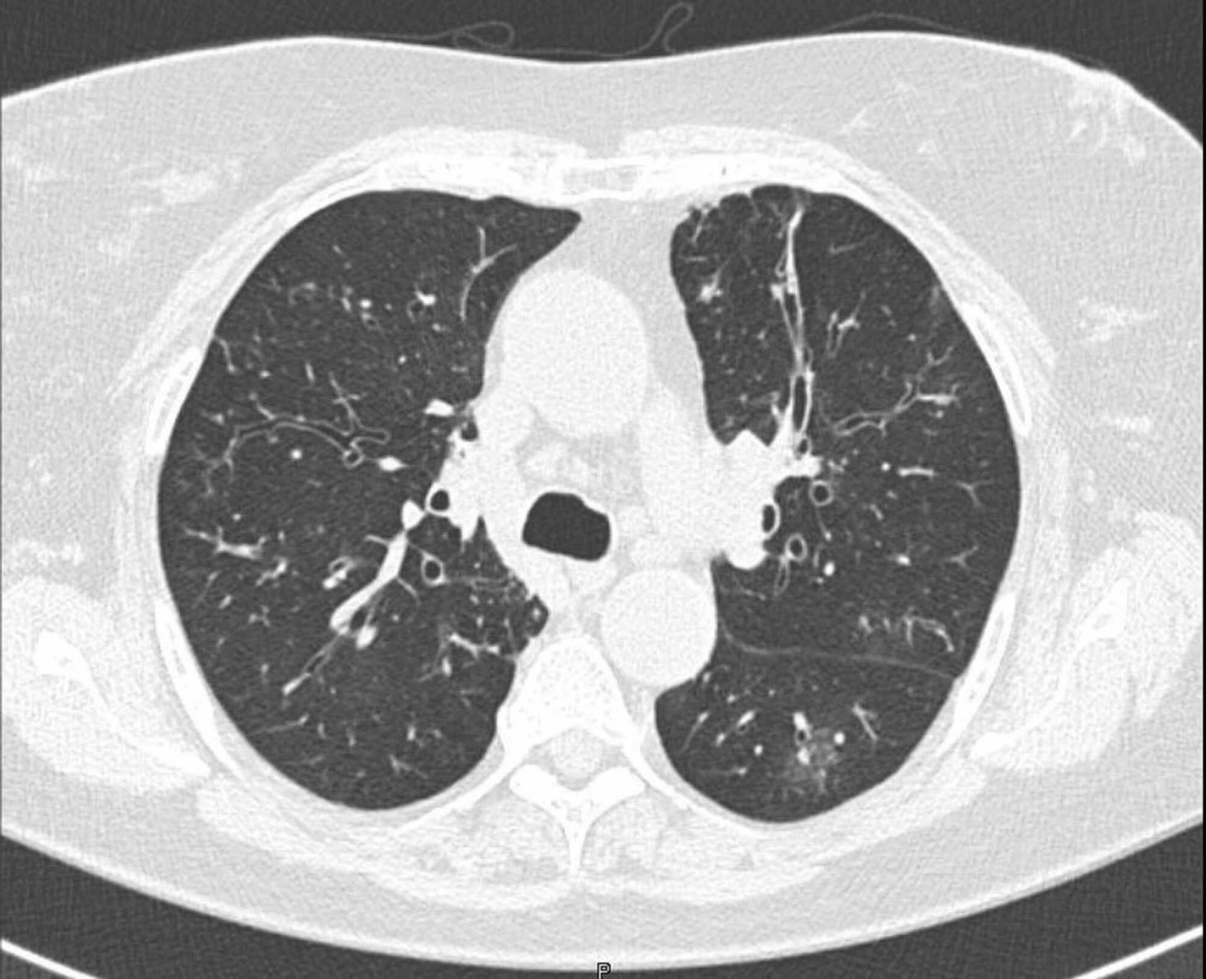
CT chest of the patient taken in August 2019 at Upstate Medical University See multifocal airspace opacities and diffuse upper lobe predominant bronchiectasis.
